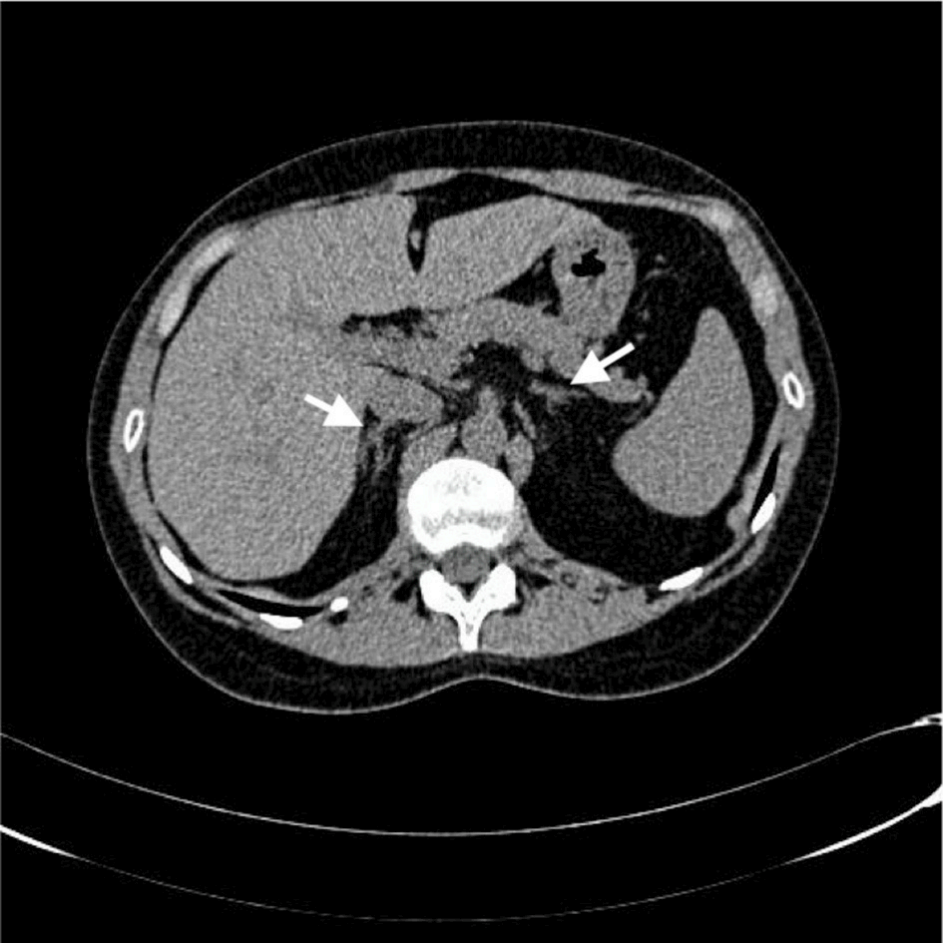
Computed tomography scan showing normal adrenal glands.
